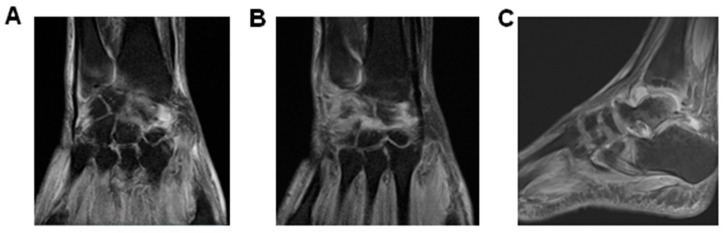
Development of severe tenosynovitis during arboviral coinfection. MRI of wrists and right ankle was performed 278 days post symptom onset to assess joint inflammatory evolvement showed in (A) (right wrist) and (B) (left wrist), synovitis and tenosynovitis bilaterally. Coronal images demonstrated the presence of edema and excessive fluid in carpus and radioulnar joints, and also in flexor and extensor compartments. (C) sagittal MRI Diffusion-Prepared (DP) weighted sequence with fat suppression image of the right ankle showing synovial overgrowth within the tibiotalar and intertarsal joints. Signs of plantar fasciitis and hyperintense abnormality were also observed, corresponding to a pattern of edema.
